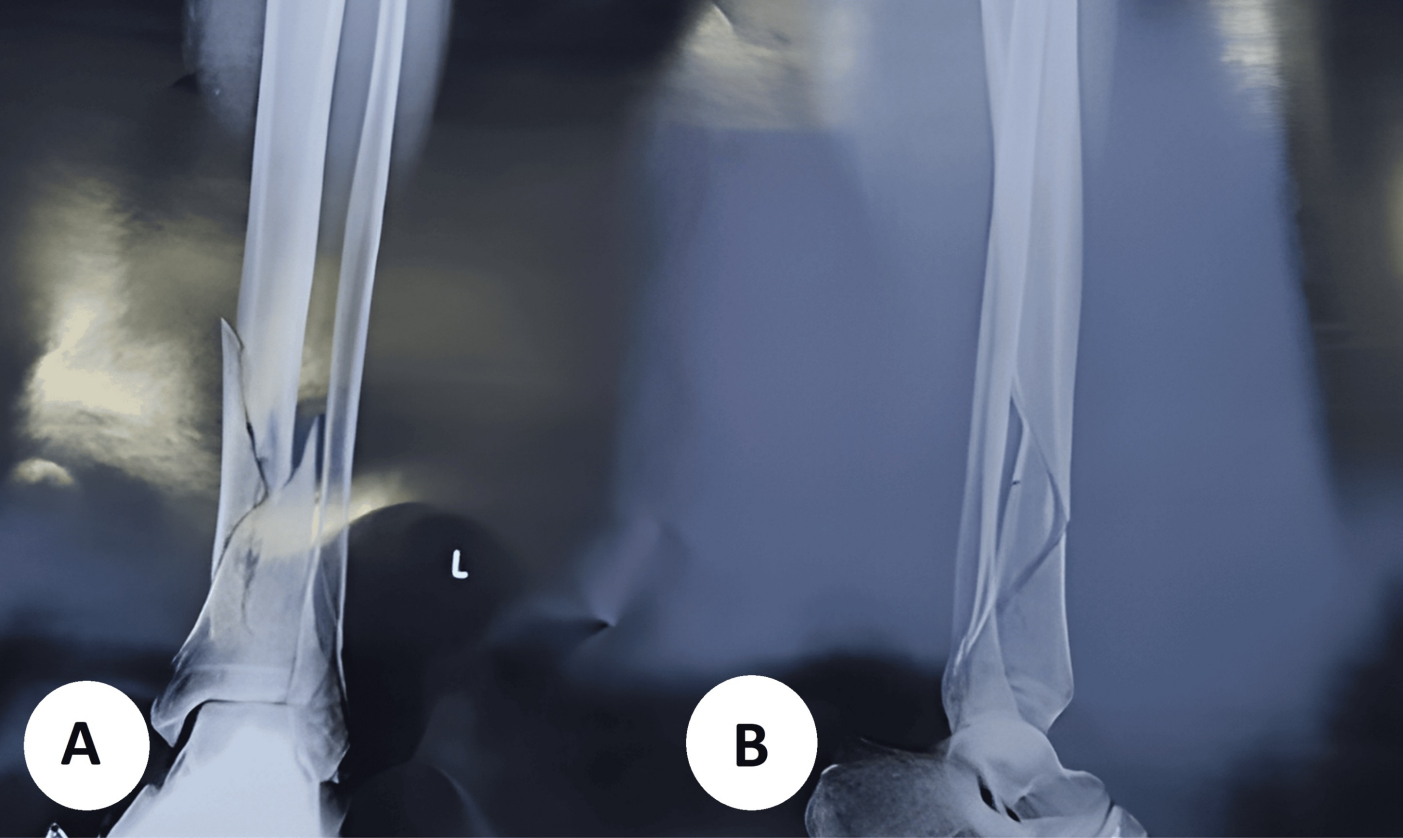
Preoperative radiographs of the left distal tibia (A) Anteroposterior view showing a displaced, extra-articular distal-third tibial shaft fracture with a long-oblique/spiral configuration and a small butterfly fragment; the ankle joint surface is preserved. (B) Lateral view confirming the oblique fracture plane with sagittal angulation and translation of the distal fragment, without evident intra-articular extension.
